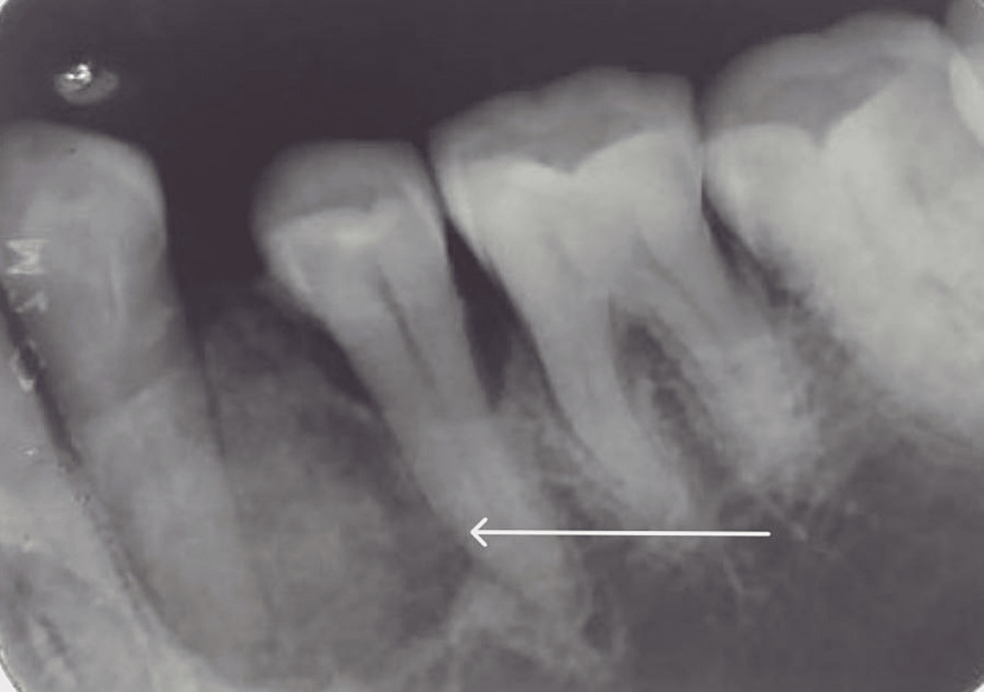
IOPA revealing a unilocular mixed lesion with an internal ground-glass appearance, a thin radiopaque margin, and concentric expansion of the affected bone involving teeth 34-35. IOPA, Intraoral Periapical Radiograph
